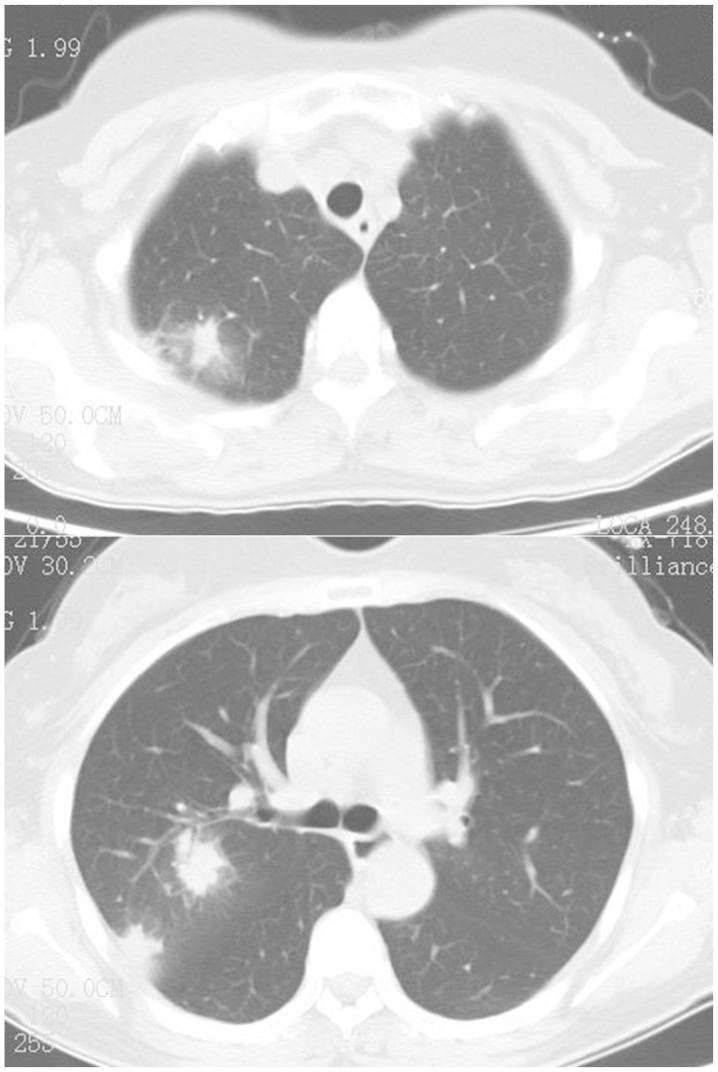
Initial chest radiography showing patchy, nodular consolidation in the right lung, and some localization in the subpleural area.
